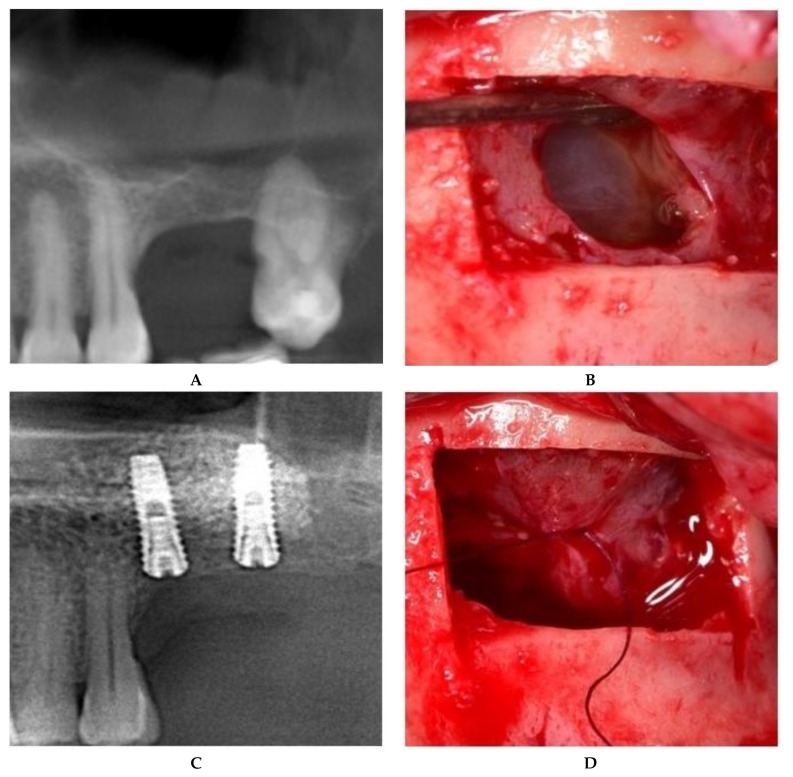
Sinus floor augmentation simultaneous with mucocele removal. (A) Preoperative CBCT of the left maxillary sinus. (B) Mucocele pseudocyst visible through the incision. (C) Suture of the incised Schneiderian membrane. (D) Panoramic image after implant placement.
